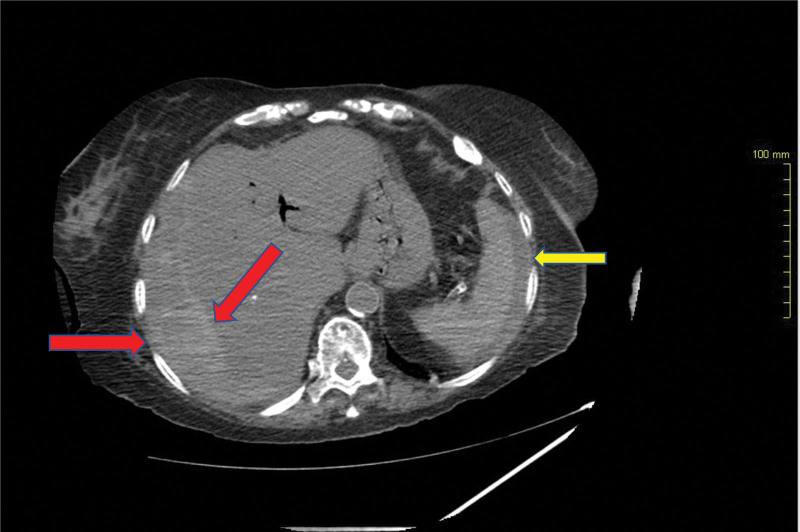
Axial view of computed tomography (CT) scan showing a subcapsular hepatic hematoma involving the right hepatic lobe (red arrows) measuring around 37 mm in maximum thickness with perisplenic fluids (yellow arrow) indicating intra-abdominal ascites.
